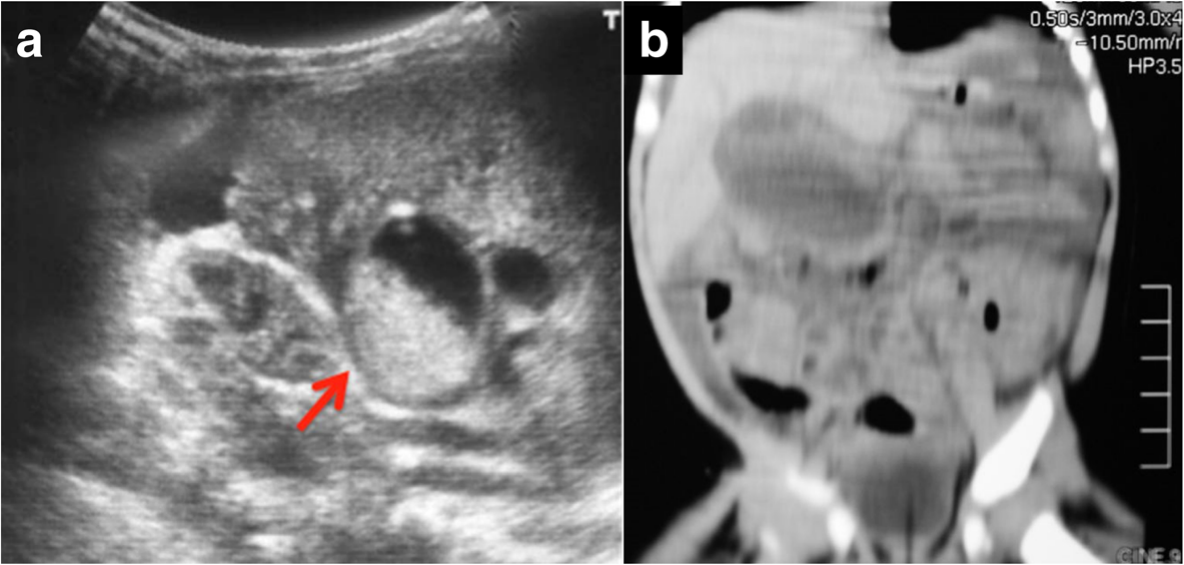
Abdominal ultrasound and CT images. Ultrasonography (Panel a) showed a cystic lesion in the hepatic hilum, with accumulated fluid and debris. CT scan (Panel b) showed extrahepatic bile duct dilatation with massive ascites
